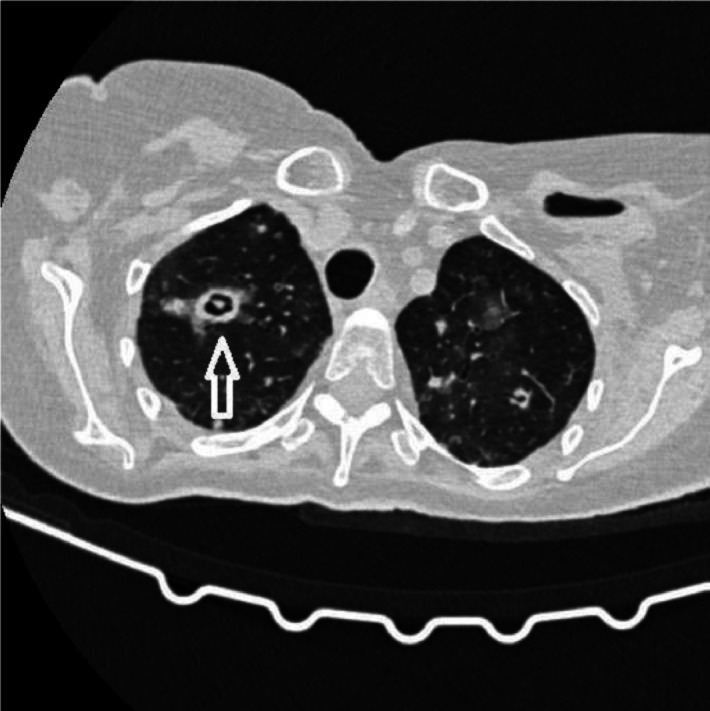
The CT scan shows an air crescent sign.
